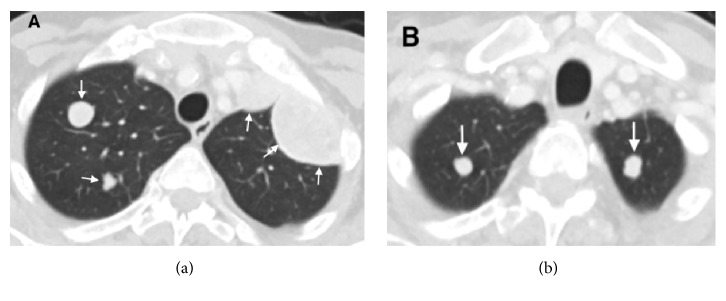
Axial contrast-enhanced computed tomography (CT) scan of chest showing bilateral multiple lung metastases (white arrows) prior to nivolumab treatment.
